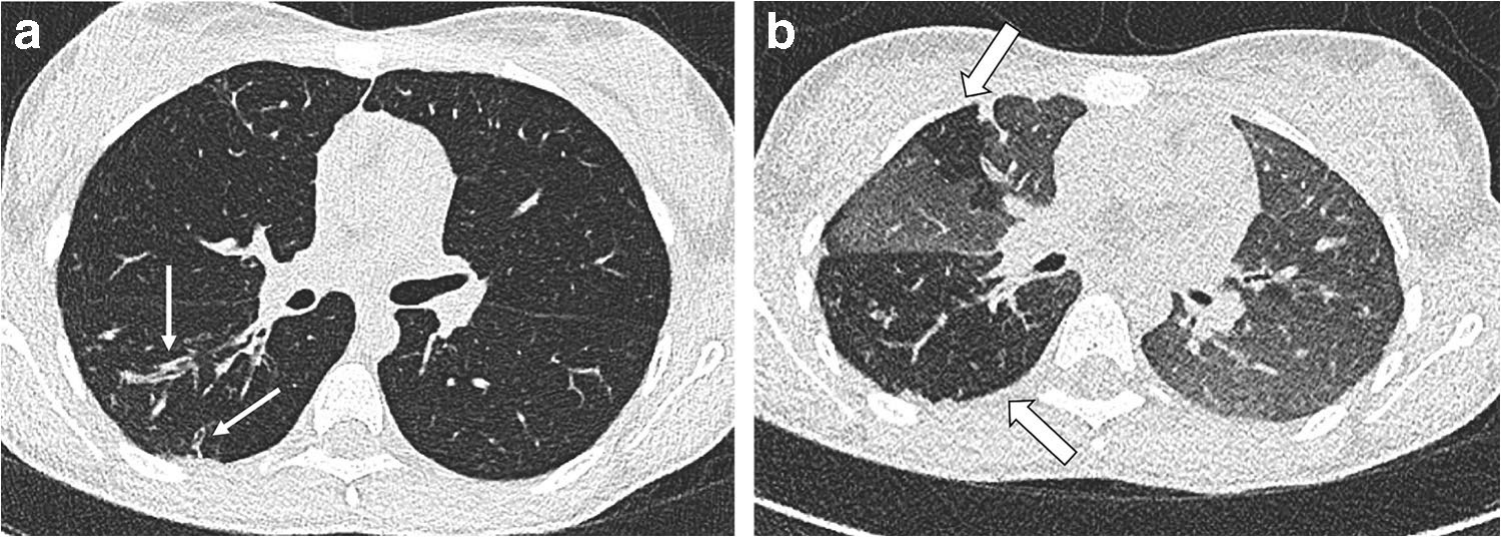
End-inspiratory and end-expiratory spirometry-guided non-enhanced CT in a 13-year-old girl with cystic fibrosis at her biennial follow-up. a, b End-inspiratory (a) and end-expiratory (b) axial CT images show clear bronchial wall thickening and bronchiectasis in the upper segment of the right lower lobe (*thin arrows*) and low-attenuation regions on the same area and also in the ventral part of the right upper lobe (*thick arrows*), indicating chronic small airways disease with trapped air and perfusion impairment
